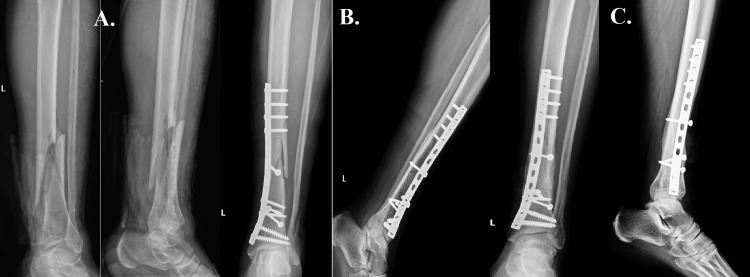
Case two A: shows a comminuted fracture affecting the lower third of the distal tibia after a traumatic injury while pivoting during a skiing accident. B: shows the alignment after the surgical reduction and fixation. C: follow-up radiographic imaging one year after surgery, shows a successful outcome with strong fracture consolidation, demonstrating the effectiveness of the surgical strategy and the patient's subsequent healing.
